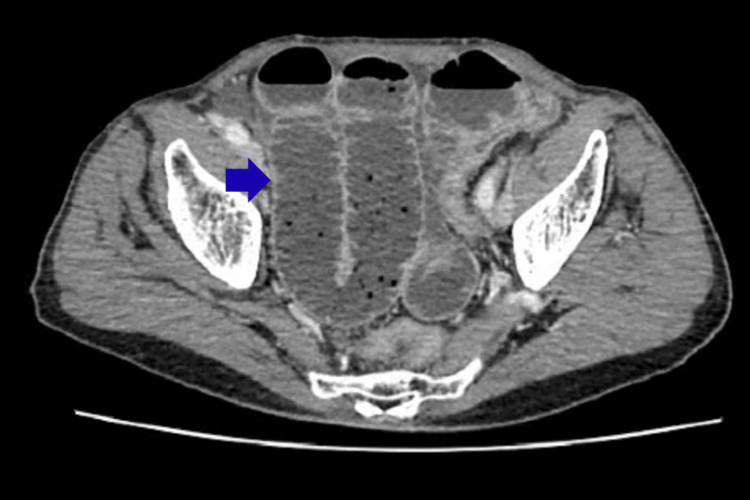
Contrast-enhanced CT (CECT) of the abdomen, axial section, demonstrating small-bowel volvulus (blue arrow).
